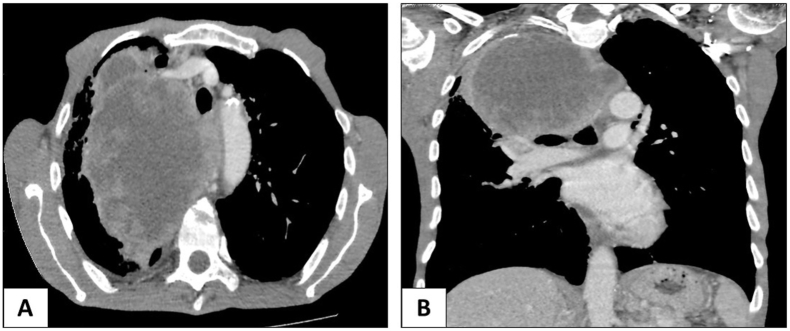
A) Chest CT scan in axial section showing right mediastino-pulmonary mass largely necrotic and measuring 13 cm in long axis. B) Coronal section showing that the mass pushes back the trachea and the esophagus and invades the carina.
